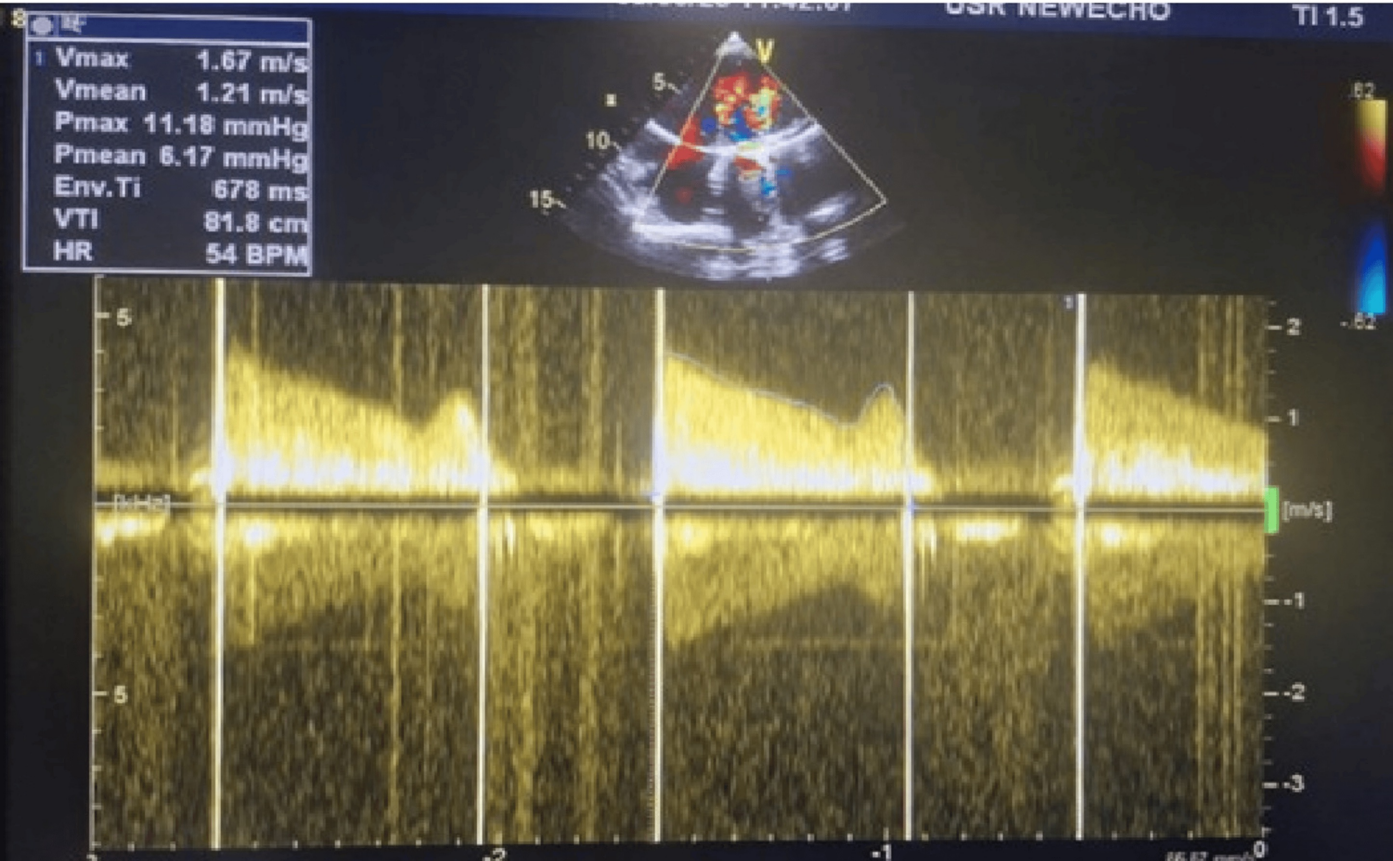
Echocardiography of the second episode of post-thrombolysis with continuous wave Doppler ultrasound Echocardiography of the second episode of post-thrombolysis with continuous wave Doppler demonstrating successful normalization of transmitral gradients (Pmax 11.18 mmHg, Pmean 6.17 mmHg). Pmax - peak gradient; Pmean - mean gradient
